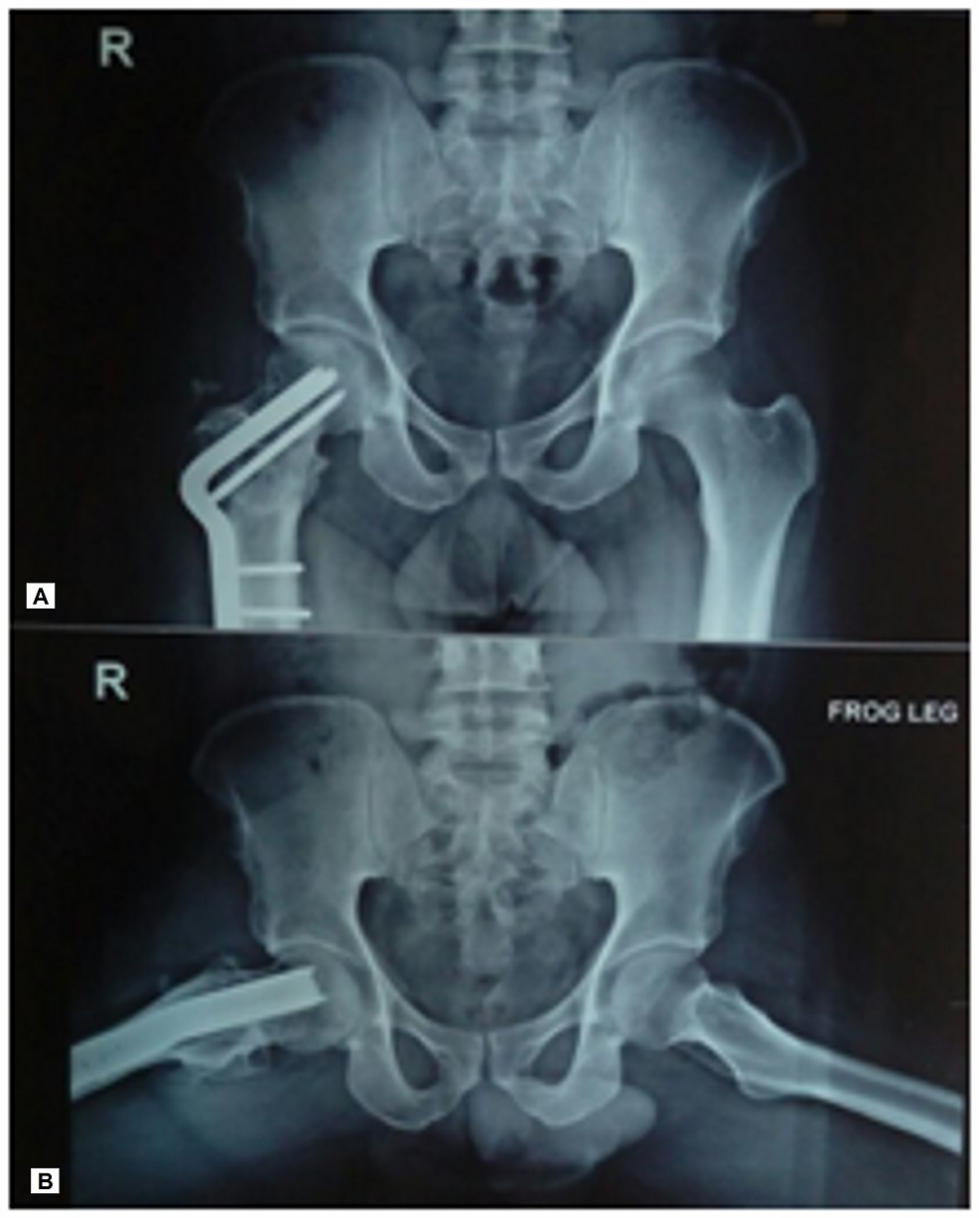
(A, B) Radiographs at four-year follow-up showing well-consolidated femoral neck with no evidence of avascular necrosis
